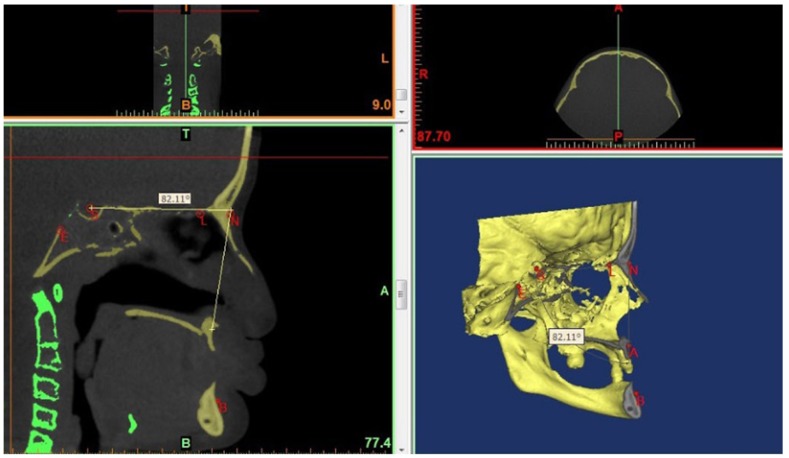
a,b. Saggital slice image for cephalometric analysiswith the Mimics program (a), 3-D image obtained with CBCT (b).
